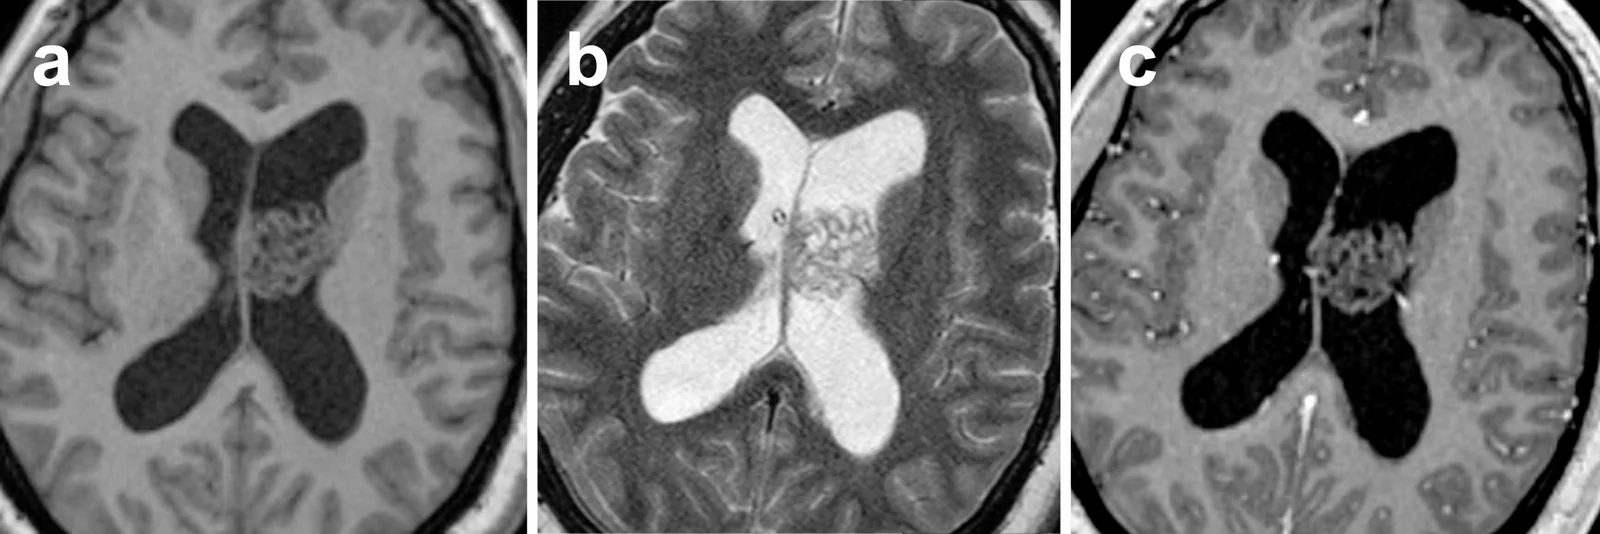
A 24-year-old man with central neurocytoma presenting with headache. Axial T1-weighted image (a), T2-weighted image (b), and contrast-enhanced T1-weighted image (c). A multilobulated “soap-bubble-like” tumor is seen near the foramen of Monro, composed of multiple cystic areas and showing minimal contrast enhancement
